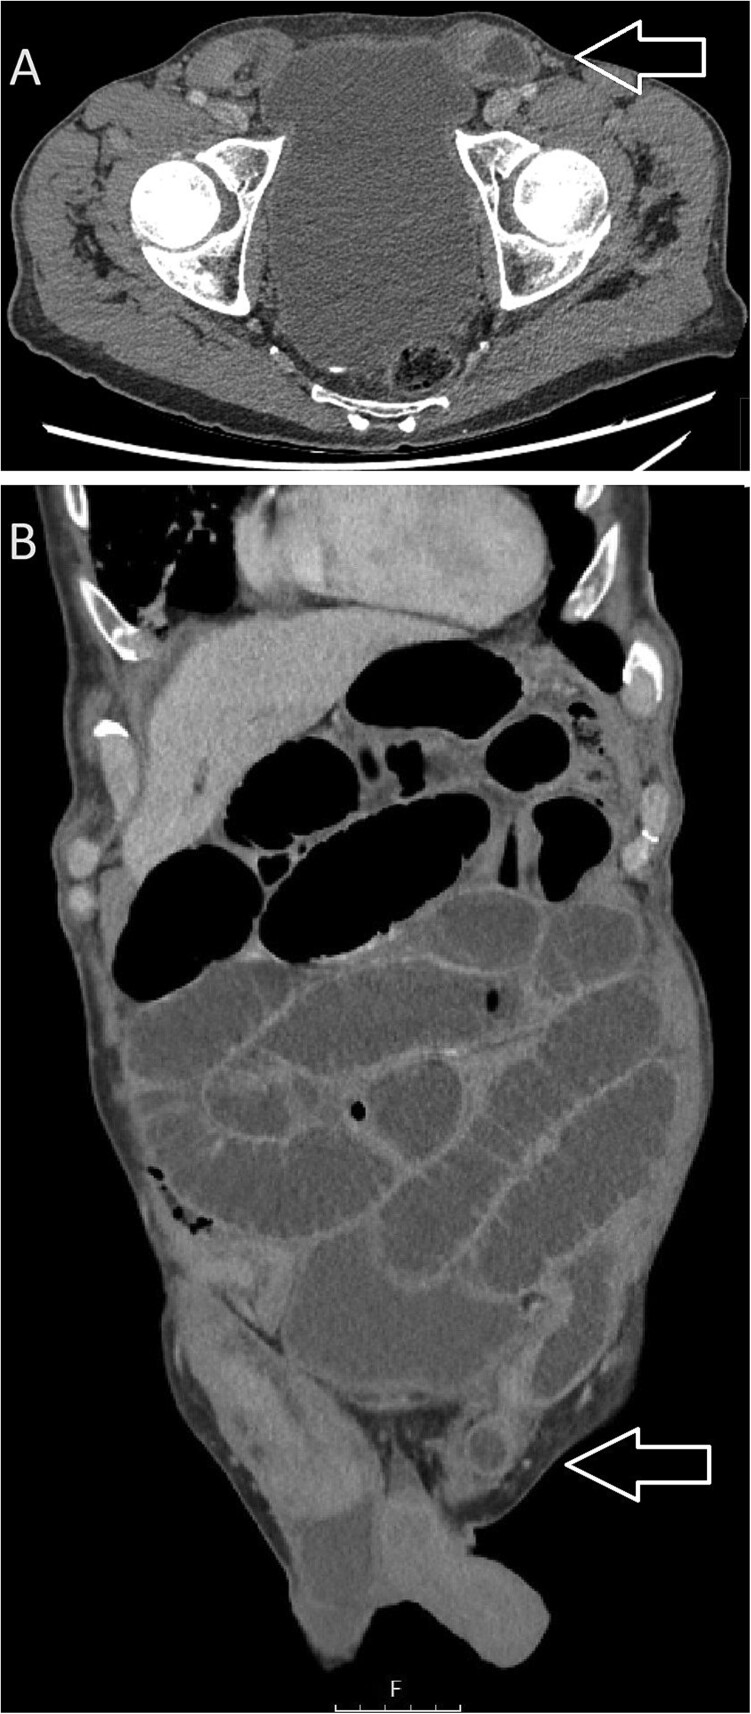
CT images: (A) axial and (B) coronal views suggestive of small bowel obstruction secondary to an obstructed, incarcerated left inguinal hernia. Arrows have been added to the image to mark the site of the left inguinal hernia.
